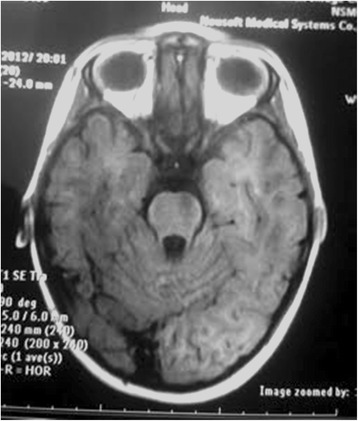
MRI image of Patient II. It indicates mild cerebellar atrophy in an axial Image of brain MRI
